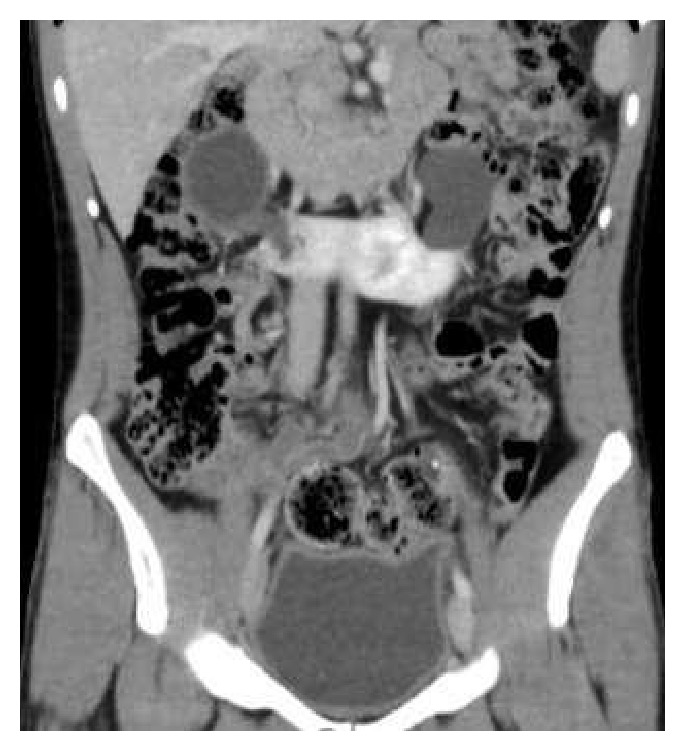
Initial preoperative CE-CT scan (coronal section) showing horseshoe kidney with right hydronephrosis.
